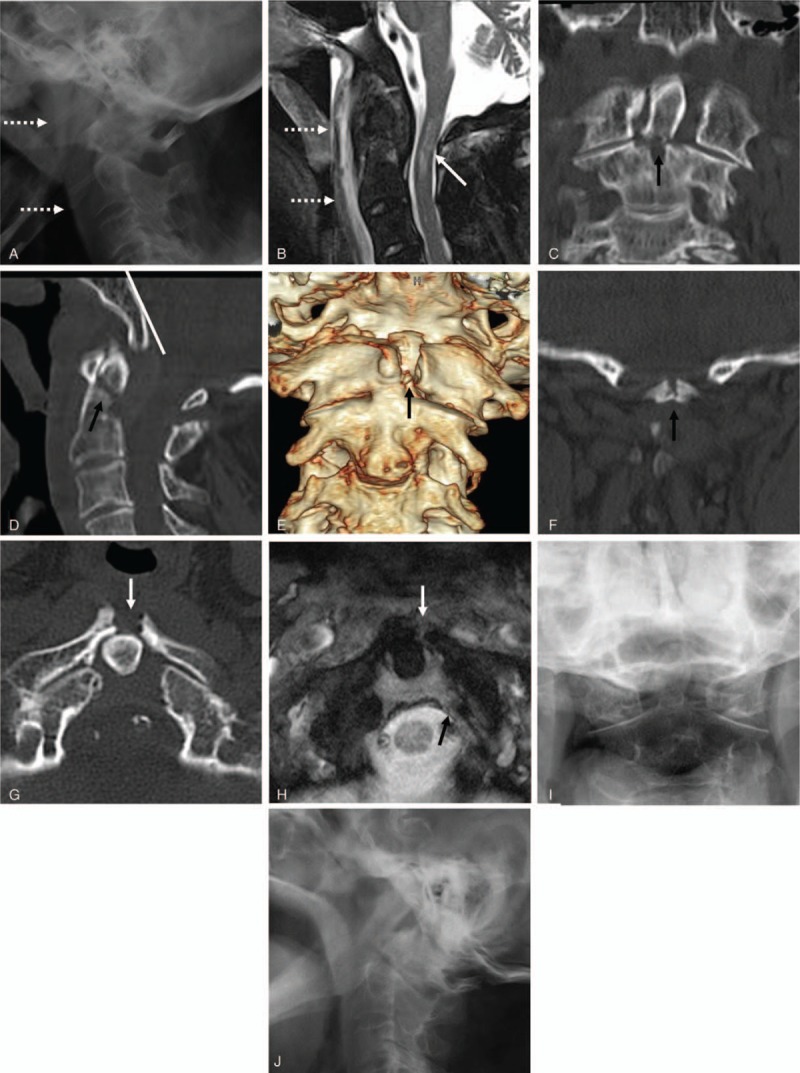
Initial lateral radiographs of the cervical spine (A) and sagittal MRI (B) showing increased anterior soft tissue swelling due to retropharyngeal hematoma (white dotted arrows) and intramedullary hemorrhage (white arrow). Coronal (C) and sagittal (D) reconstructed CT scans demonstrated running of the Wackenheim line (white line) behind the tip of the dens, indicating posterior AOD and type II dens fracture (Anderson-D’Alonzo classification) (black arrows). Three-dimensional (E) and coronal (F) reconstructed CT scans showing a midline sagittal split fracture of the C1 anterior arch (white arrows). Axial CT scan (G) and MRI (H) revealed a midline sagittal split fracture of the C1 anterior arch (white arrow) and a type II TAL injury according to Dickman's classification. Three months following conservative treatment, the posterior AOD and type II dens fracture were posteriorly displaced compared to the initial presentation (I and J). AOD = atlantooccipital dislocation, CT = computed tomography, MRI = magnetic resonance imaging.
